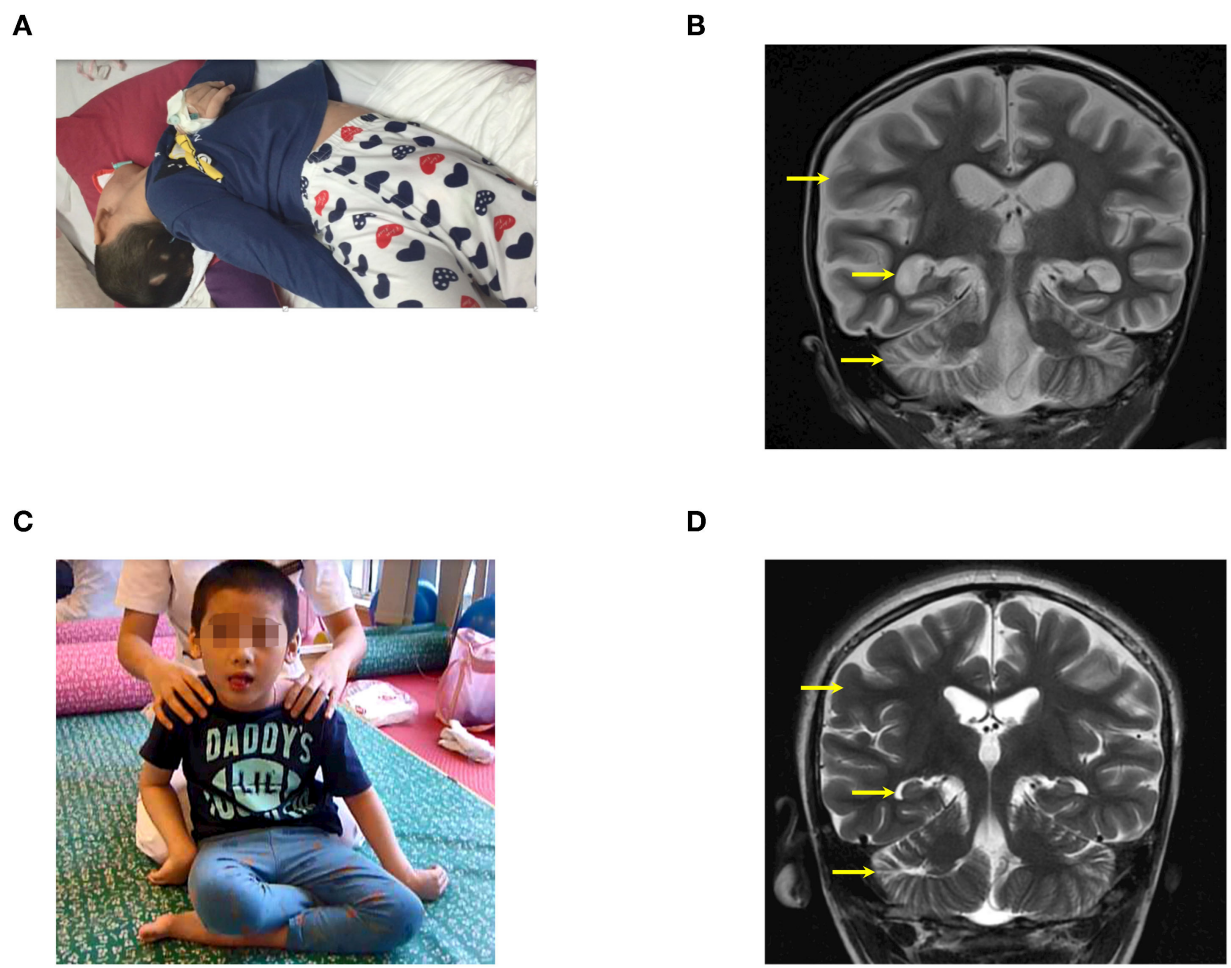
The patient (case 3) before and after autologous BMMNC transplantation. (A) The patient before BMMNC transplantation is in a VS 4 months after the drowning. He had no awareness and suffered from quadriplegia with muscle spasticity at level V. (B) Diffuse cerebral atrophy at the supratentorial and infratentorial regions on brain MRI before transplantation (arrows). (C) Six months after the third transplantation, the patient could respond to calling, recognized objects, sit up, and expressed feelings. (D) Brain MRI showed a remarkably reduced cerebral atrophy with near-normal cerebral parenchyma (arrows) after stem cell transplantation.
